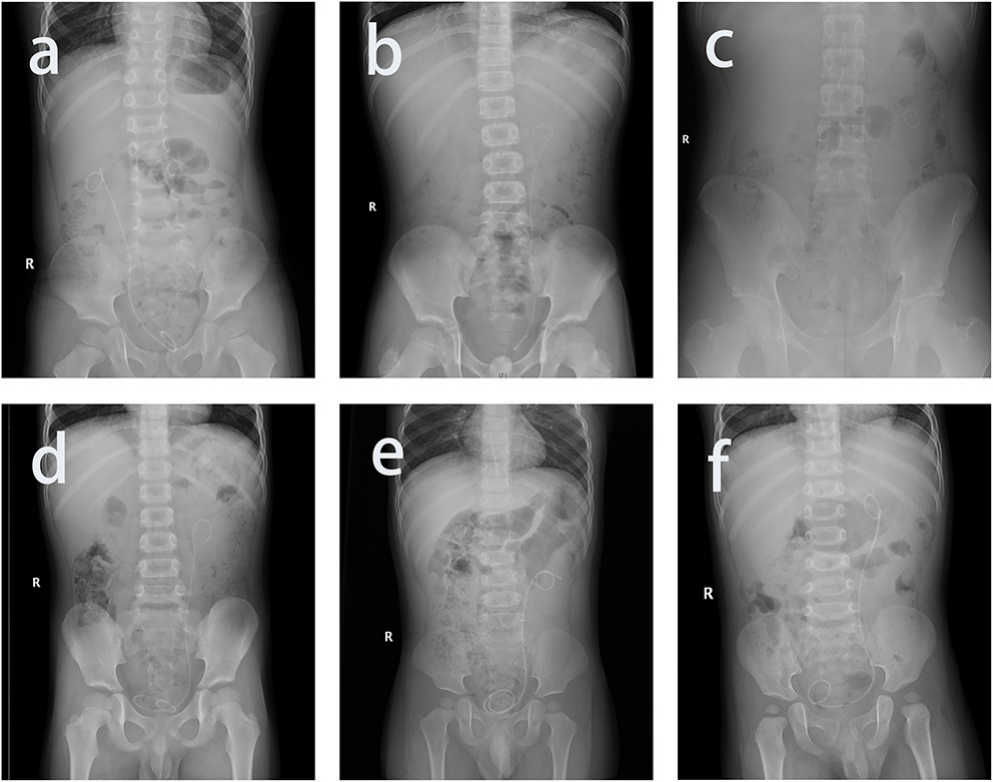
The possible reason for failed transurethral retrograde fishing the double J urethral stent in kidney–ureter–bladder radiography manifestation. (a) The ideal model with satisfactory position, the lower double J urethral stent (JJS) bent into a coil, and the direction of the coil crosses the anterior–posterior body in some way; (b) lower JJS been almost straight, paralleled anterior–posterior body; (c) JJS reached right bladder wall cross the trigone of bladder from the left; (d) JJS folded in the trigone of bladder; (e) the coil of lower JJS was much more broad than usual and high folded in the bottom of bladder; (f) bladder capacity was large, and the coil of lower JJS broadened a lot.
